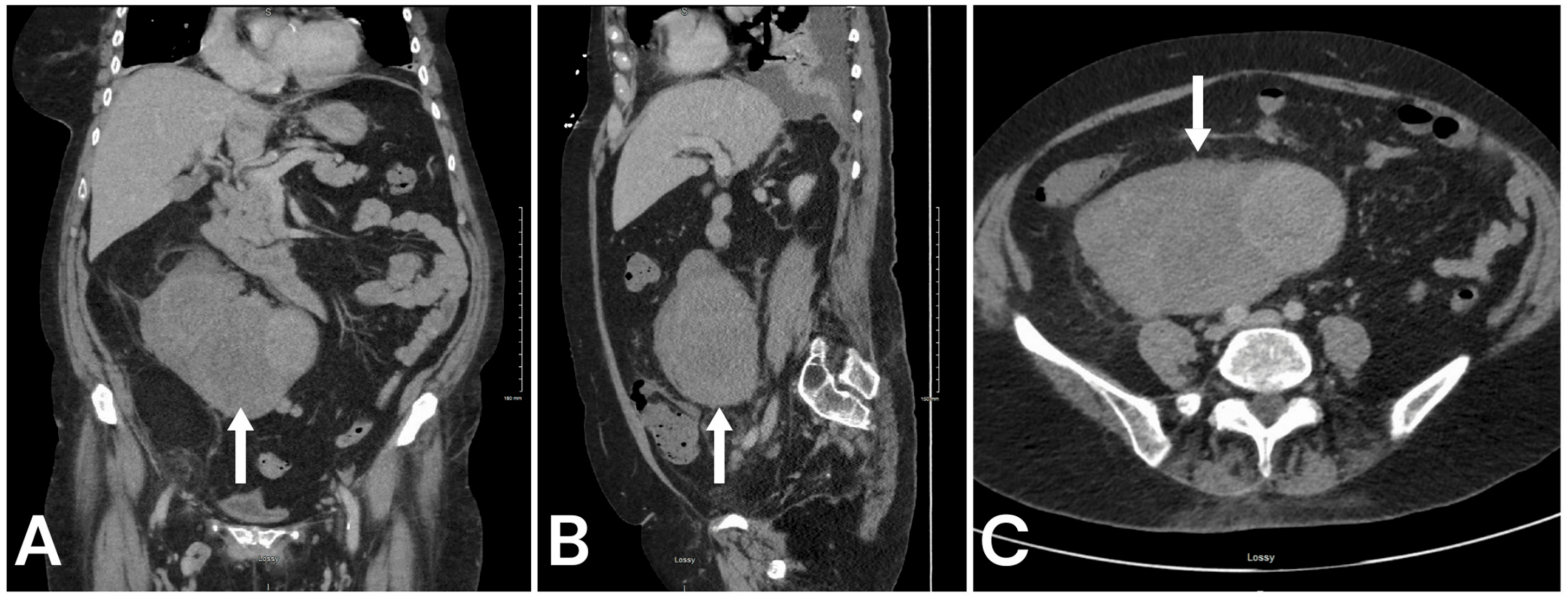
CT imaging with contrast demonstrating heterogeneous enhancement of the large mass (white arrows) located within the retroperitoneum and attached to the mesentery of the right colon. This appeared contiguous with the abdominal vein. No adenopathy or other enhancing lesions noted and no liver metastases. (A) Coronal. (B) Sagittal. (C) Axial.
